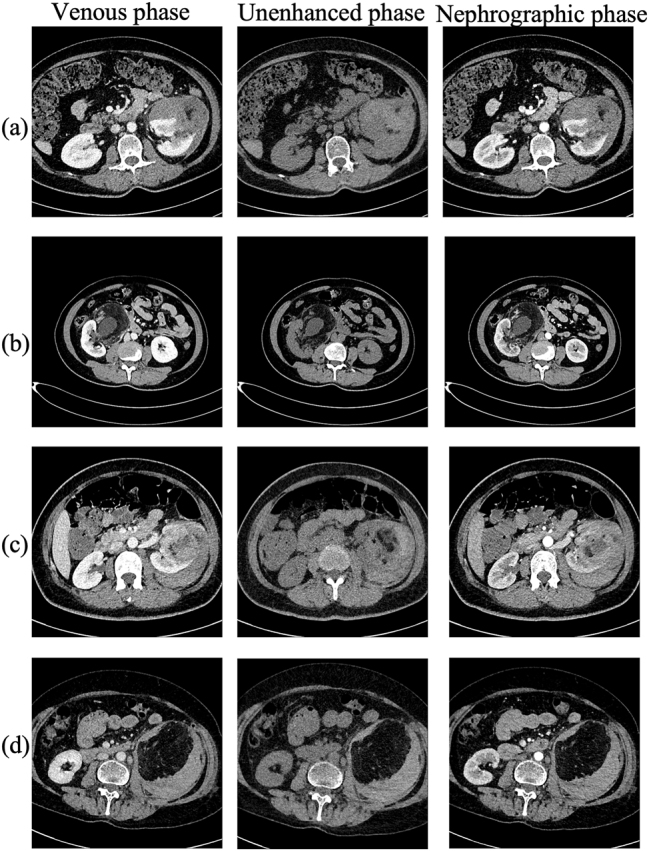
Representative contrast-enhanced CT images of hemorrhagic AMLs with capsular rupture. Axial CT images from four different patients with hemorrhagic renal angiomyolipoma (panels a–d). For each patient, representative slices from the unenhanced, corticomedullary (arterial), and nephrographic (venous/contrast-enhanced) phases are presented. (a) A ruptured AML showing high-attenuation intratumoral hemorrhage and perirenal hematoma. (b) A large exophytic AML with capsular disruption and active contrast extravasation during the enhancement phase. (c) A fat-poor AML with mixed intratumoral densities and subcapsular bleeding. (d) A posteriorly located AML demonstrating heterogeneous enhancement and perirenal fluid collection extending along Gerota’s fascia. These cases highlight the phase-dependent CT manifestations of hemorrhagic rupture, including intratumoral bleeding, capsular breach, and perirenal hematoma formation.
